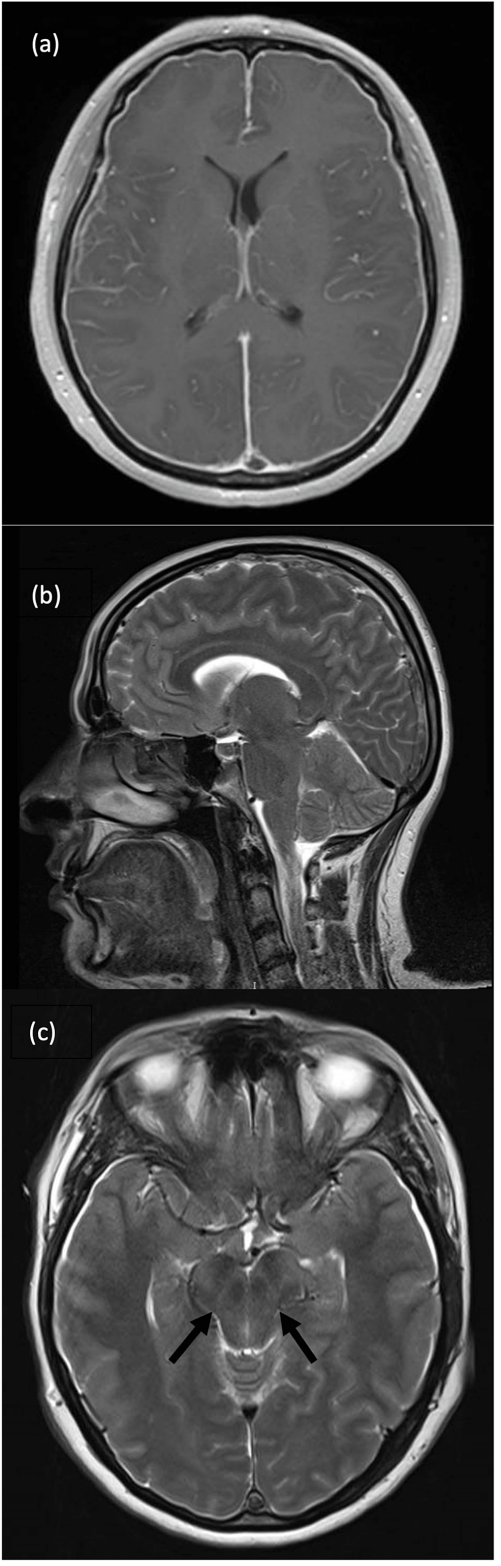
(a) Contrast-enhanced brain MRI, axial T1, showing diffuse cerebral convexity dural thickening and pachymeningeal enhancement, (b) Brain MRI, sagittal T2, showing low-lying cerebellar tonsils and enlarged pituitary gland, (c) Brain MRI, axial T2, showing effacement of the basal ambient cisterns (arrows)
